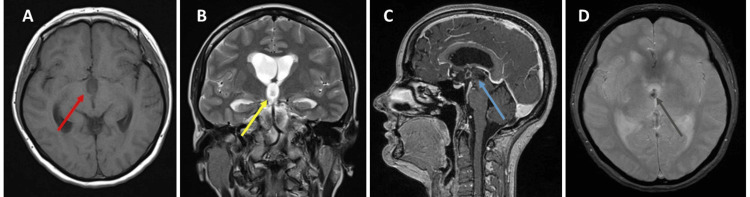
Preoperative MRI - Hypointense in T1 (red arrow) and hyperintense in T2 (yellow arrow) (A and B); T1 with contrast showed a mild dotted enhancement (blue arrow) in the center of the lesion (C); Hypointense signal on the gradient-echo (T2*) (black arrow - D).
